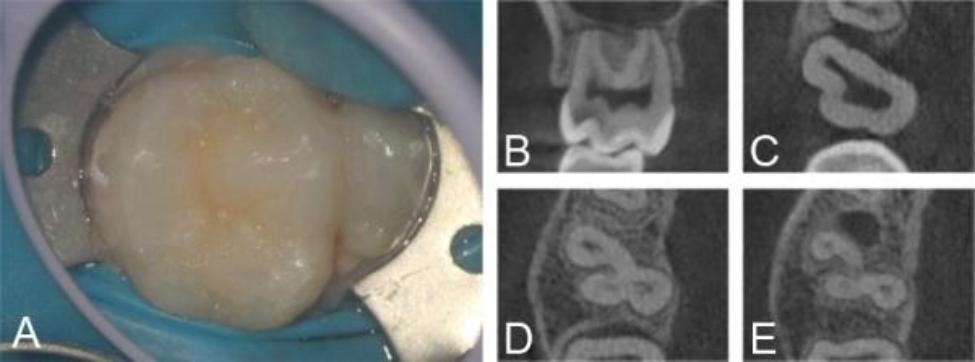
Preoperative intraoral photographs and radiographs of the geminated tooth in case 1. A: On occlusal intraoral view, an abnormal extra cusp is clearly visible on the buccal side of the crown, distinct from the crown of the right maxillary first molar by a sulcus. B: CBCT coronal cross-sections reveal a radiolucency that has already extended toward the distopulpal horn. C-E: CBCT axial cross-sections confirm that the diseased tooth shares a common root and root canal system with the supernumerary tooth
